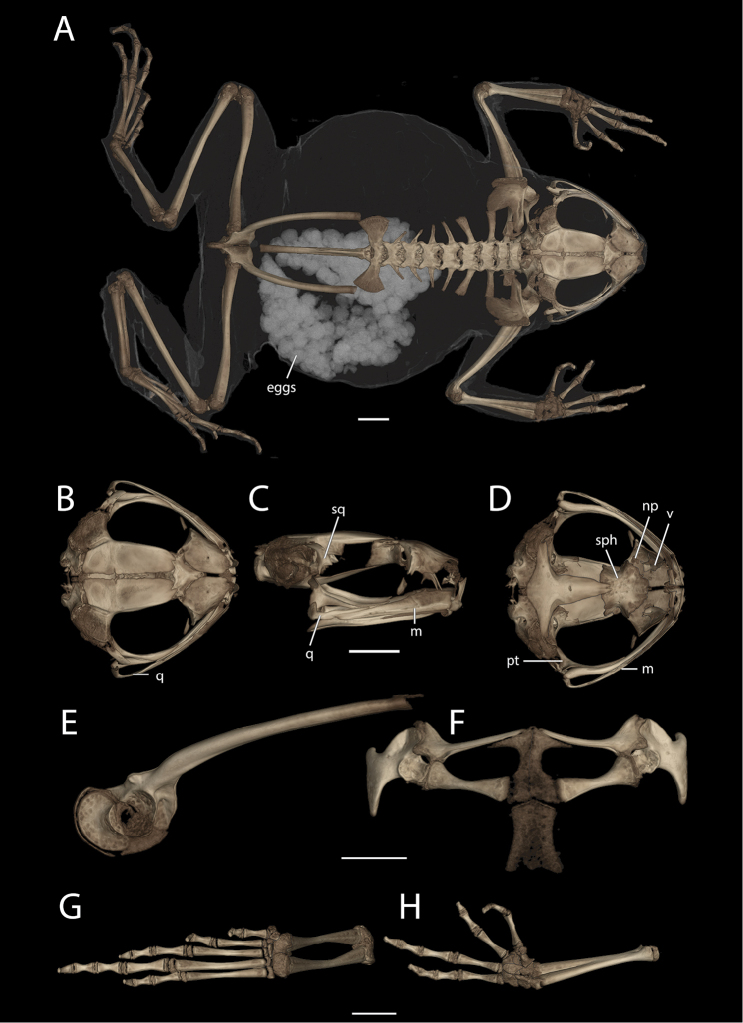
High-resolution Computed Tomography reconstructions showing the skeleton of the holotype (UF 184184) of *Poyntonophrynuspachnodes* sp. n. in dorsal view (A), skull in dorsal (B), right lateral (C) and ventral (D) views, pelvis in right lateral view (E), pectoral girdle in ventral view (F), and right foot (G) and right hand (H) in ventral views. Selected skeletal elements are labeled based on discussion in the text. Panel A also highlights the eggs that are visible within the oviducts. Abbreviations: m – maxilla; np – neopalatine; pt – pterygoid; q – quadrate; sph – sphenethmoid; sq – squamosal; v – vomer. Scale bars in each row equal 2 mm.
